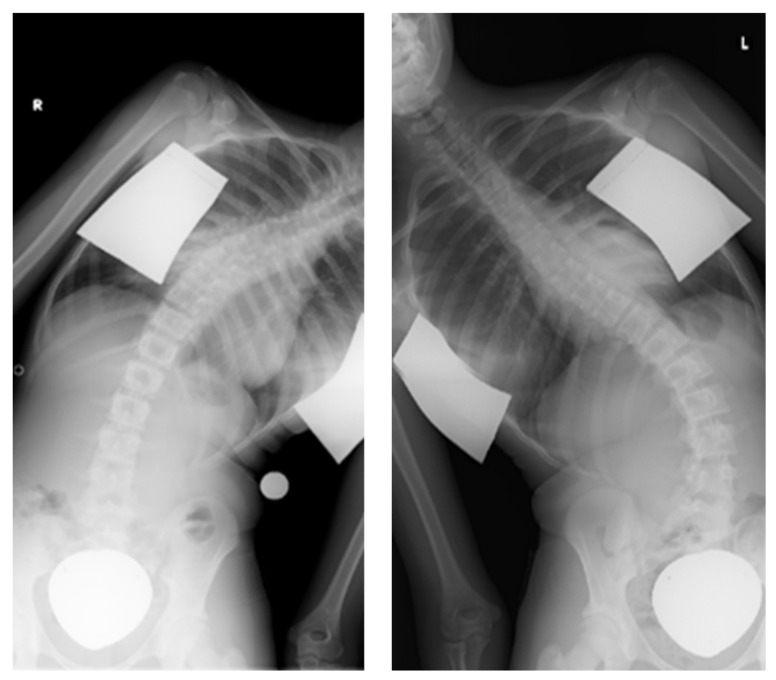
Preoperative major curve bending radiographs.
